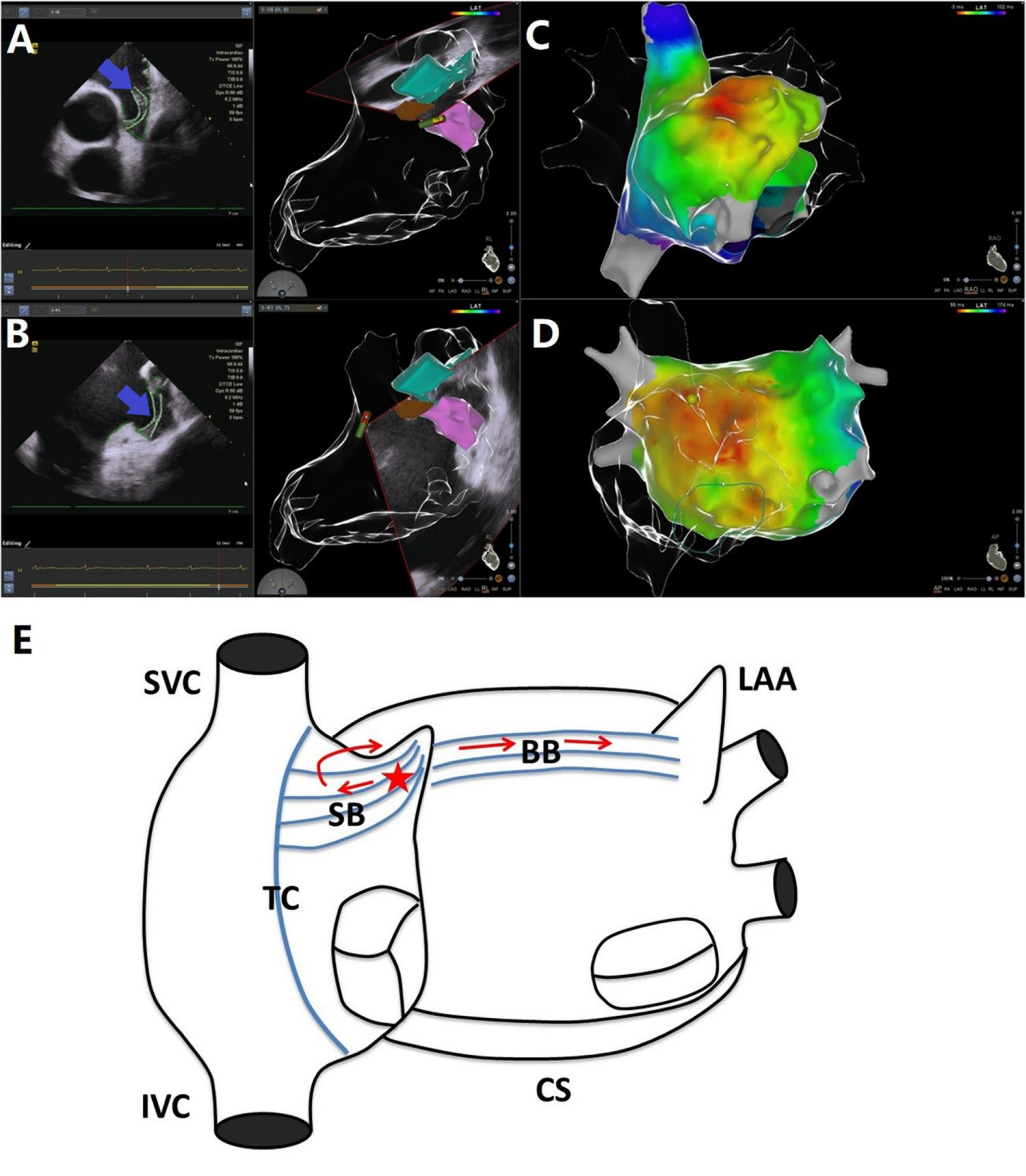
A, B Structures in the right atrial appendage under intracardiac echocardiography. The blue arrows indicate the sagittal bundle.
